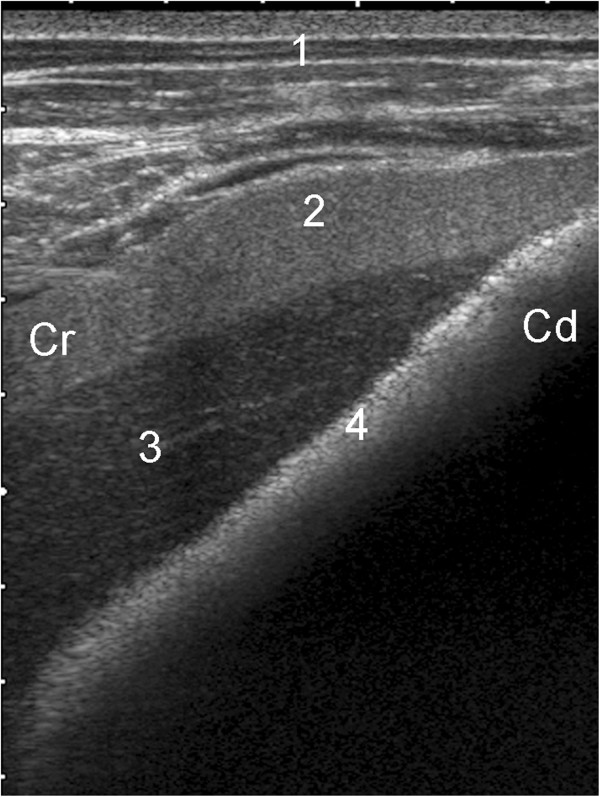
Ultrasonogram of the spleen and liver. Ultrasonogram of the spleen and liver in a 61-day-old Holstein-Friesian calf viewed from the ventral midline. 1 Ventral abdominal wall, 2 Spleen, 3 Liver, 4 Reticulum, Cr Cranial, Cd Caudal. (depth of the image = 8 cm).
